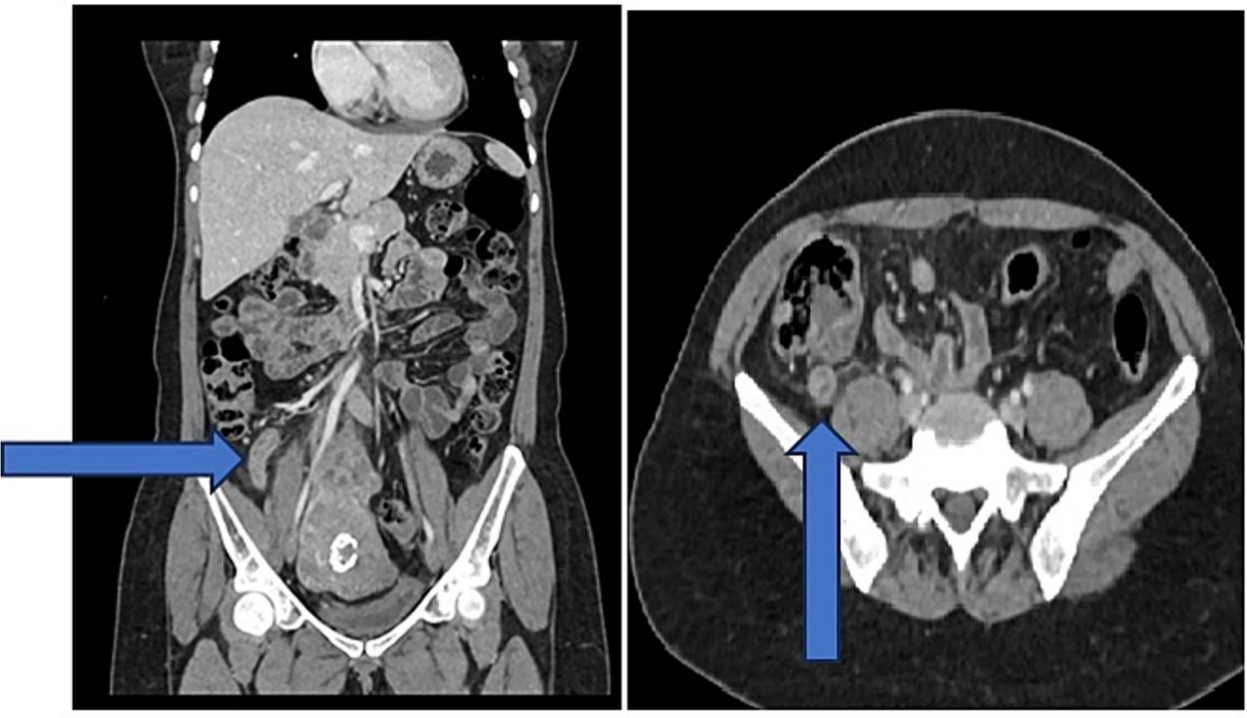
CT images with blue arrow pointing to inflamed appendix.
